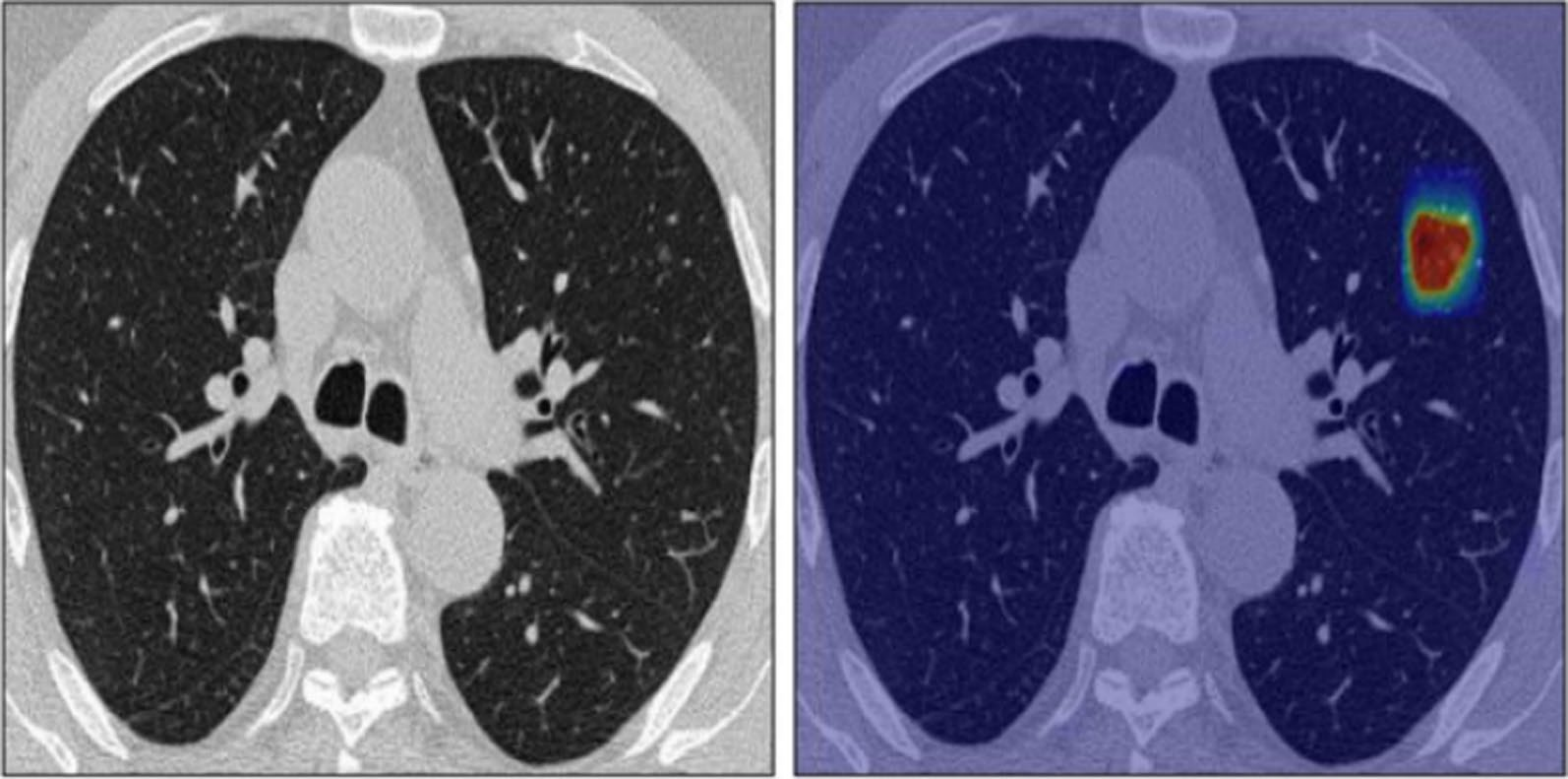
A 67-year-old male patient presented with fever and dry cough. CT chest study was initially unremarkable and after doing color-coded images an ill-defined left upper lobar faint small ground glass nodule became more obvious
